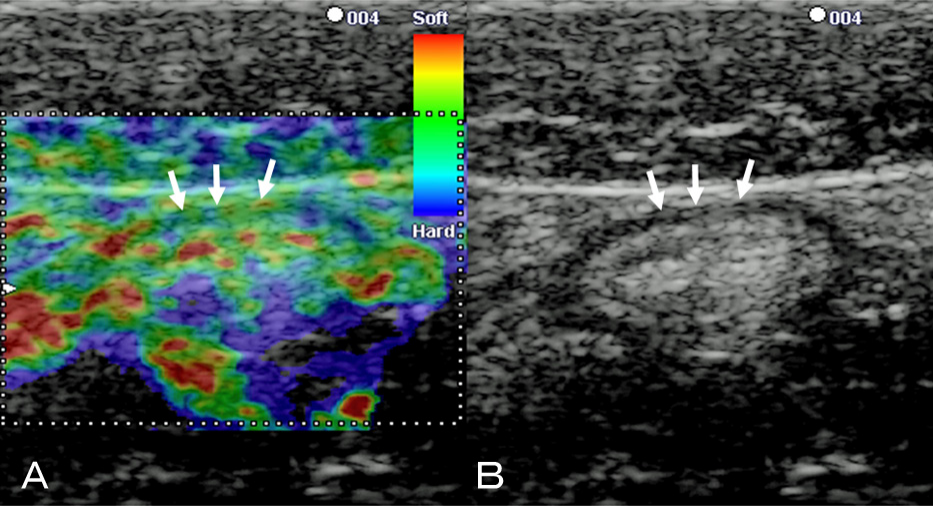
B-mode ultrasound and corresponding EG images of the normal colon wall. A: Corresponding EG of the normal wall shows almost all parts of the wall appear green (arrows)..B: B-mode ultrasound image of the normal colon wall shows 5 layers (arrows).
